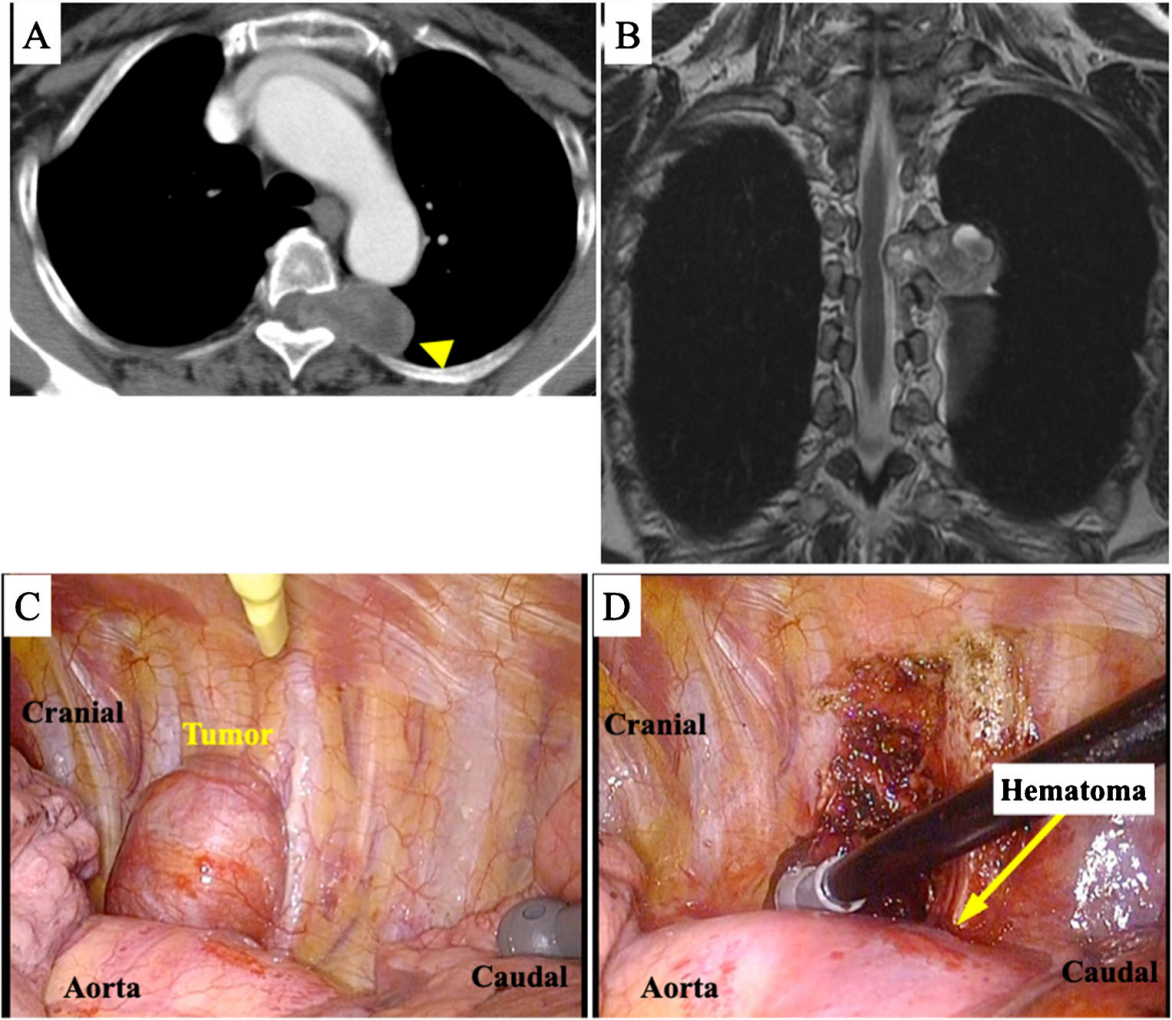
Images of patient 1. A and B The tumor was located in the left posterior mediastinum and was suspected to extend to the spinal canal through the fourth thoracic (Th4) intervertebral foramen on computed tomography (CT) and magnetic resonance imaging (MRI). C and D Intraoperative photographs. The descending aorta concealed the vertebral side of the tumor. The forceps contacted the aorta, which resulted in a pleural hematoma
